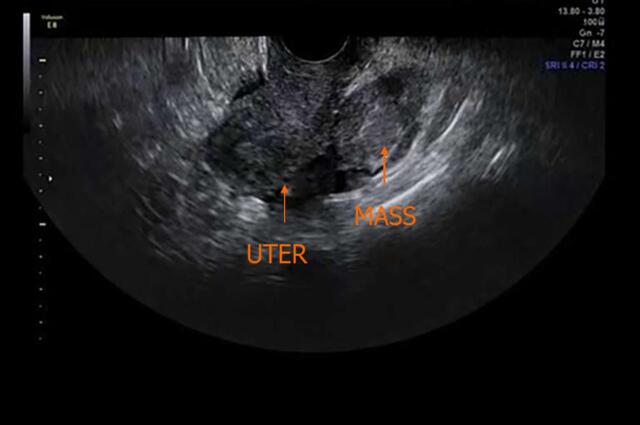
Image of ultrasound examinations.
